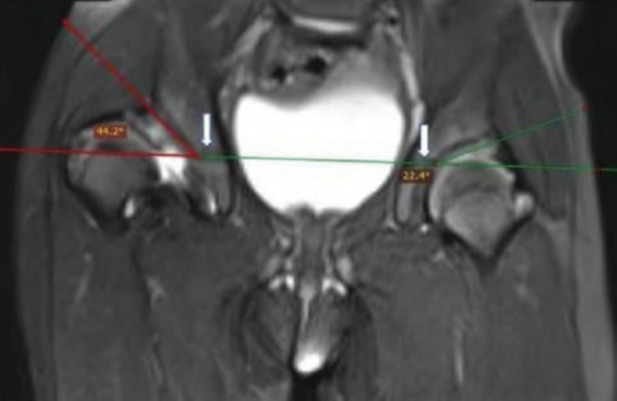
Cartilaginous Acetabular index (CAI): Coronal T2-weighted fat-suppressed image of the hip with right-sided DDH in a 1-year-old female child. The acetabular index is calculated by an angle formed by a line passing through superior aspect of triradiate cartilage (arrows) i.e., Hilgenreiner’s line, and a second line along the acetabular roof. The right acetabular index (44.2°) is increased as compared to the normal left side (22.4°).
